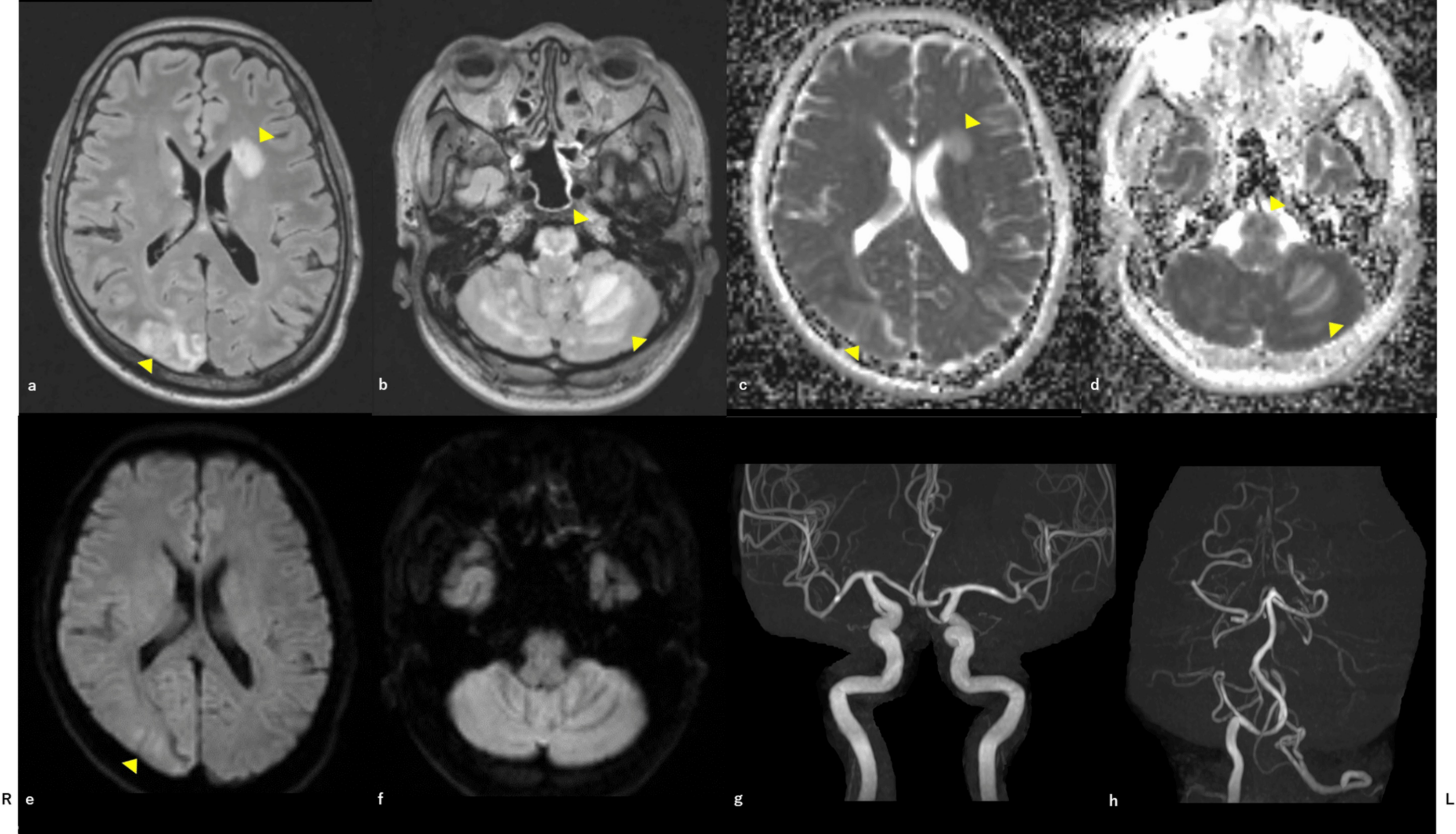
Brain MRI on admission. Axial FLAIR (a, b) and ADC maps (c, d) of the hemisphere. There were hyperintense lesions in the right parieto-occipital lobe, left basal ganglia, and white matter around the lateral ventricles, brainstem, and bilateral cerebellar hemispheres. Axial DWI of the hemisphere (e, f). Some of the lesions are high intensity. Brain magnetic resonance angiography revealed no significant intracranial stenosis of the main trunk artery (g, h). MRI: magnetic resonance imaging; FLAIR: fluid-attenuated inversion recovery; ADC: apparent diffusion coefficient; DWI: diffusion-weighted image.
